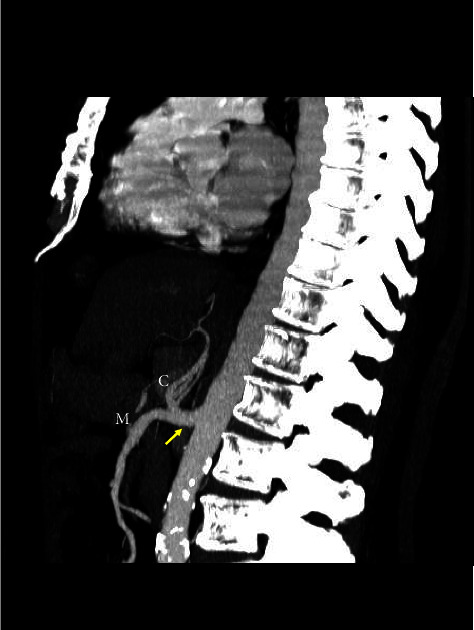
Three-dimensional volume rendering CT image showing a complete celiacomesenteric trunk (CMT) inclusive of mesenteric (M) and celiac (C) ramifications.
